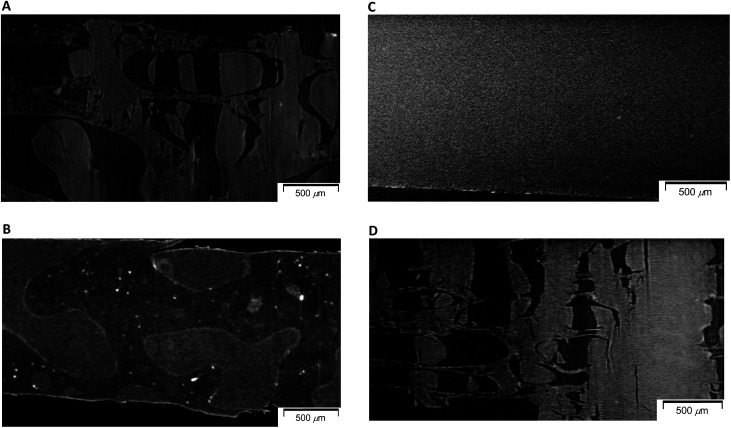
Micro-focus X-ray CT images of 50% plasticized PHA/50% PCL blends. (A) Without compatibilizers after quasi-static test. (B) Without compatibilizers after impact test. (C) With PERHEXA25B after quasi-static test. (D) With Modiper A4400 after quasi-static test.
